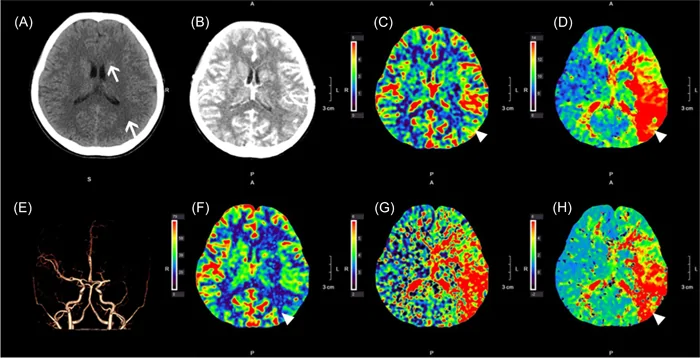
Brain imaging revealed a low‐density lesion in the left basal ganglia and parietal lobe (A, white arrowhead) with patchy, slight enhancement (B). CT perfusion imaging and CT angiography indicated a mild decrease in cerebral blood flow (CBF), prolonged time to peak (TTP), and mean transit time (MTT) in the corresponding region. The local and distal branches of the left middle cerebral artery (M1 segment) were not visualized (C–H, white triangles).
